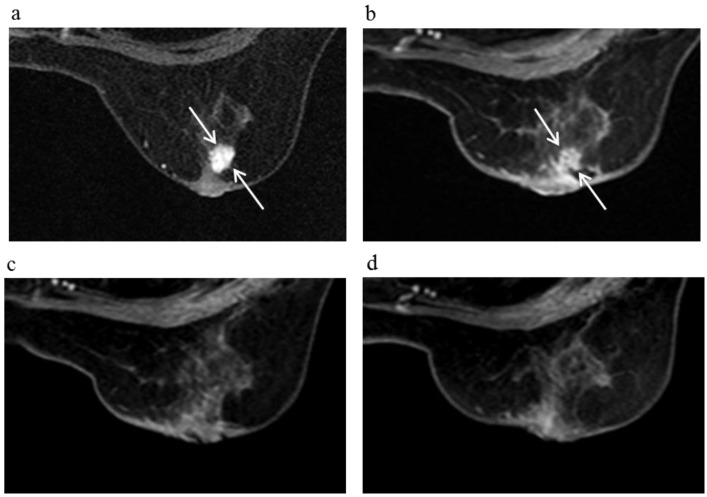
A 59-year-old female (case 18 in Table 1, Table 2 and Table 3) with right breast cancer (cT1cN0M0). CE breast MRI (a) revealed a breast tumor before KORTUC II therapy, the region of tumor was 11 mm (arrows). 6 months after the completion of KORTUC II, the tumor does not still disappear on breast CE MRI ((b): arrows); However, after 18 months (c) and 30 months (d), a complete response was achieved and no recurrence was identified on CE breast MRI.
